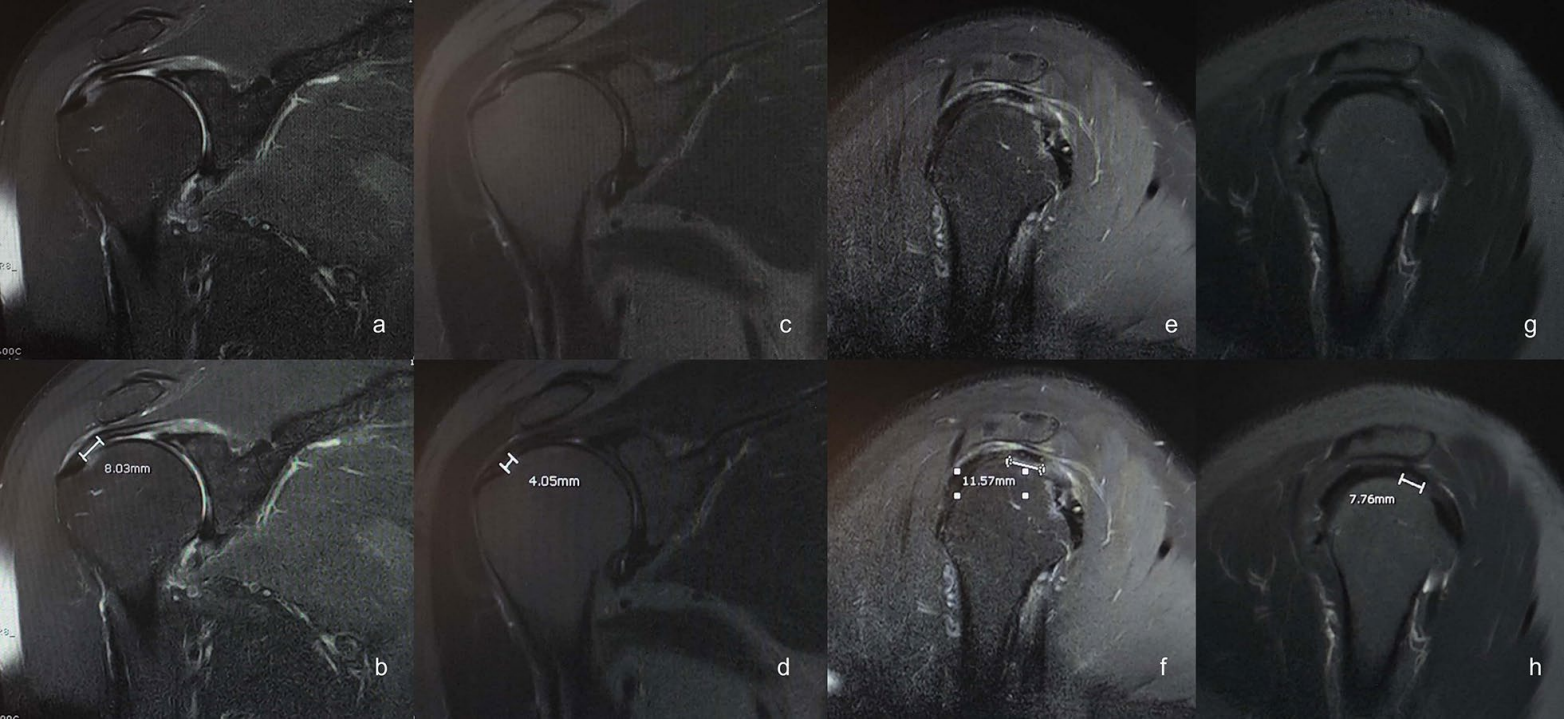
A coronal oblique MRI image of the right supraspinatus tendon tear (a) with measurement (b) before the injection. A coronal oblique MRI image of the right supraspinatus tendon tear 6 months after the injection (c) with measurement (d). A sagittal oblique MRI image of the right supraspinatus tendon tear (e) with measurement (f) before the injection. A sagittal oblique MRI image of the right supraspinatus tendon tear 6 months after the injection (g) with measurement (h)
